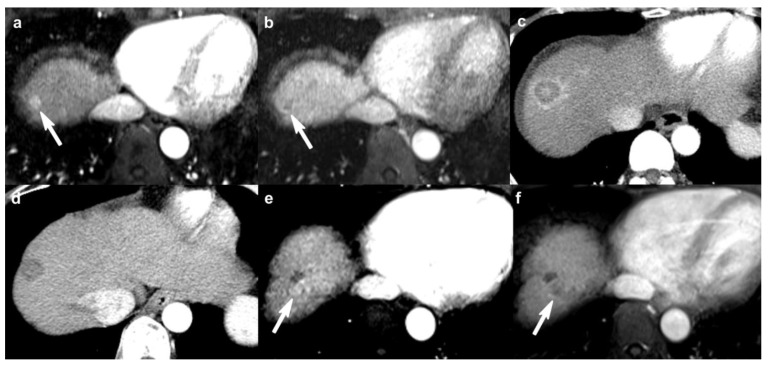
A 54-year-old man with HCC treated with RFA. (a,b) Pretreatment MR images on the arterial phase (a) and portal venous phase (b) show a 1.0-cm arterial enhancing and washout nodule on liver segment VIII dome (arrows), suggestive of HCC. (c) Immediate post-RFA CT image shows complete ablation of HCC, surrounded by expected post-treatment thin rim enhancement on the arterial phase. (d) Follow-up (after 3 months) CT image on the arterial phase shows no lesional enhancement in treated observation. This treated observation was assigned as LR-TR nonviable. (e,f) However, follow-up (after 3 years) MR images on arterial phase (e) and portal venous phase (f) show nodular arterial enhancement and washout at posterior margin of treated observation (arrows). This treated observation was assigned as LR-TR viable category and confirmed as viable HCC on explant pathology. HCC, hepatocellular carcinoma; RFA, radiofrequency ablation; MR, magnetic resonance; CT, computed tomography; LR-TR, Liver Imaging Reporting and Data System treatment response.
